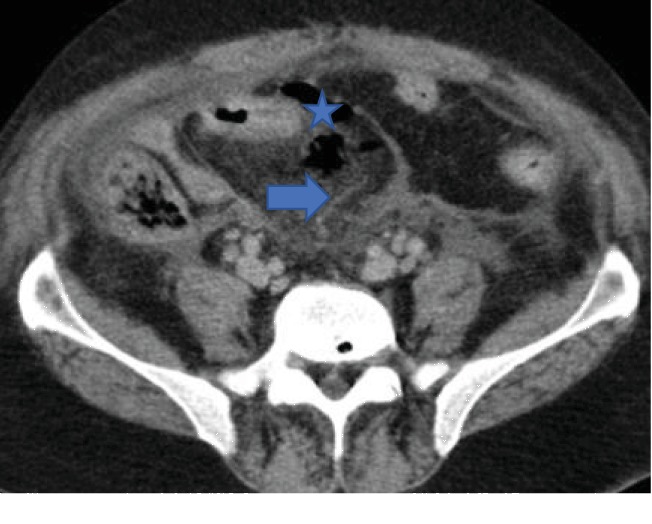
Axial CT image of the abdomen and pelvis showing pericolic gas (asterisk) and fat stranding (arrow).
